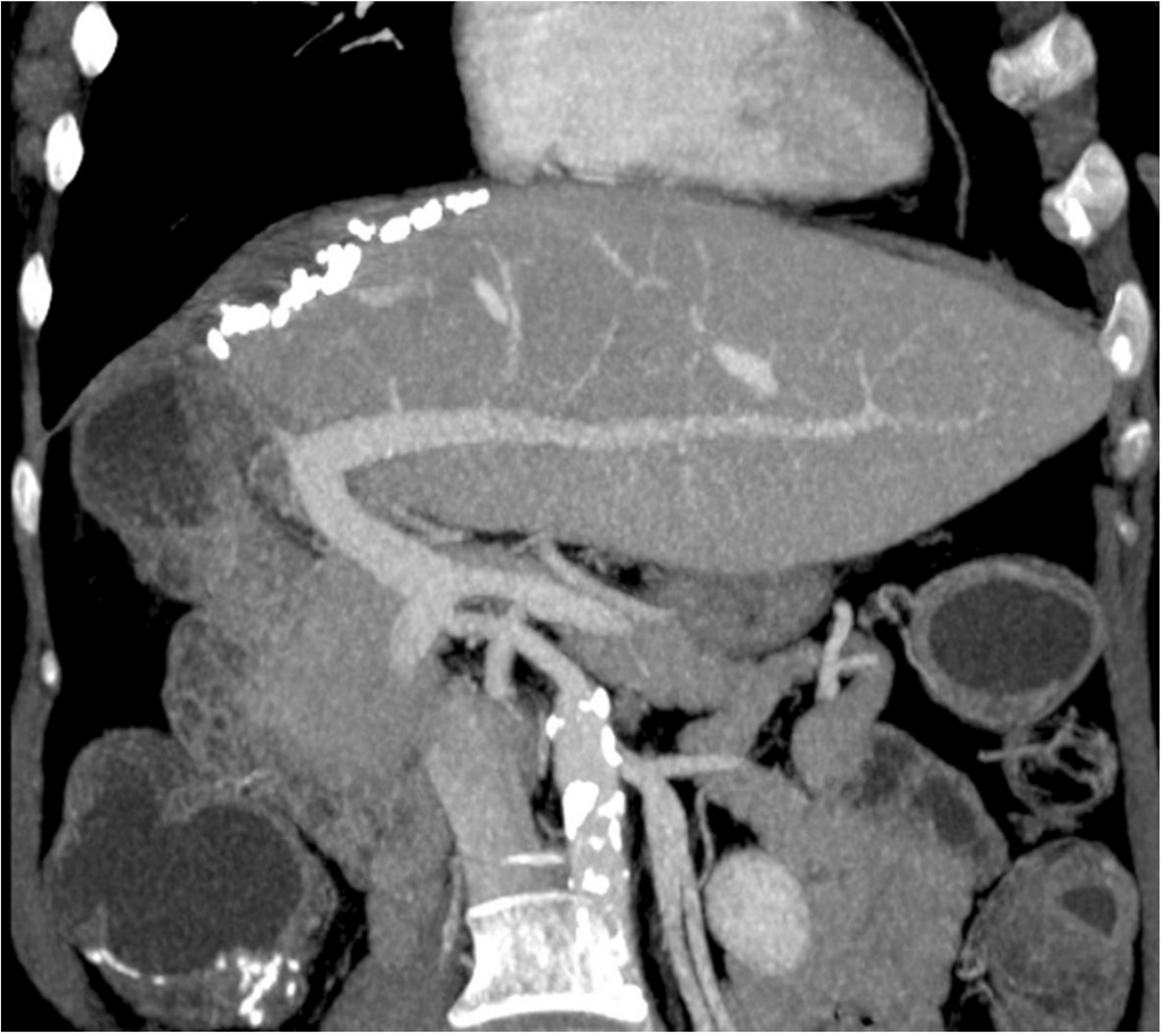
Liver surgery. MIP image from portal venous phase CT shows right hepatectomy findings: section and ligation of the right PV at its origin; shift of left liver toward the right with horizontalization of PV; prominent caliber of left PV and hypertrophy and rounded contours of the remaining liver
